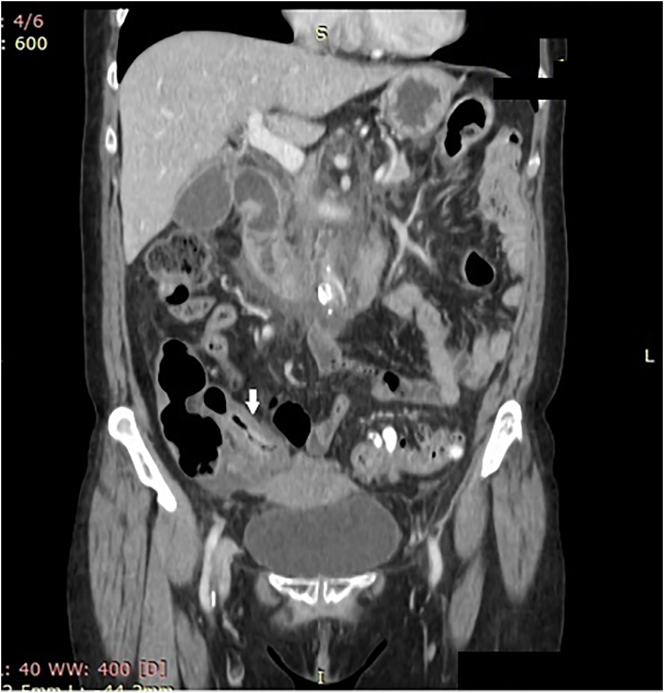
CT scan of abdomen and pelvis showing diffuse, fusiform thickening of the terminal ileum (arrowed) and enhancement of the luminal surface representing inflammation.
